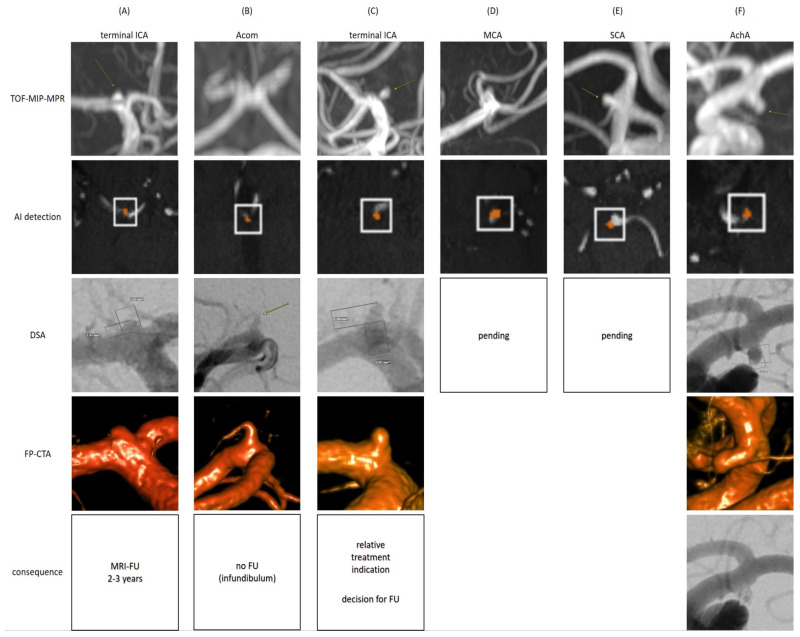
Catheter Angiography Workups of Newly Detected Findings Suspicious for Intradural Aneurysms. (A): A patient in her/his fifties with a TOF-MRA suspicious for an initial terminal ICA aneurysm who favored a catheter angiography over cMRI follow-up after consultation. The DSA demonstrated an initial aneurysm of 1–2 mm without indication for treatment. The finding will be followed up by cMRI at multi-year intervals. (B): A patient in her/his sixties with an inconclusive TOF-MRA finding at the Acom who favored catheter angiography over cMRI follow-up after consultation. The finding turned out to be an infundibulum without any necessity for further follow-up. (C): A patient in her/his thirties with a small terminal ICA aneurysm of approx. max. 2 mm as confirmed by catheter angiography. According to neurosurgical and neuroradiological consultations, there is a relative treatment indication. The patient decided to reduce risk factors by stopping smoking and will have cMRI follow-ups in a multi-year interval. (D): A patient in her/his thirties with a possible MCA aneurysm was scheduled for catheter angiography (pending/postponed for patient-specific reasons). (E): A patient in her/his sixties with a possible SUCA aneurysm was scheduled for catheter angiography (pending/postponed for patient-specific reasons). (F): A patient in her/his fifties with a saccular AchA aneurysm max. approx. 3.4 × 1.8 mm (dome height × neck width) as confirmed by catheter angiography. The aneurysm was treated by coil occlusion without any complications. Abbreviations: f—female; m—male; yo—years-old; ICA—internal carotid artery; Acom—anterior communicating artery; MCA—middle cerebral artery; SCA—superior cerebellar artery; AchA—anterior choroidal artery; TOF-MIP-MPR—time-of-flight maximum-intensity-projection multi-planar reconstruction; AI—artificial intelligence; DSA—digital subtraction angiography; FP-CTA—flat-panel computed tomography angiography; FU—follow-up.
